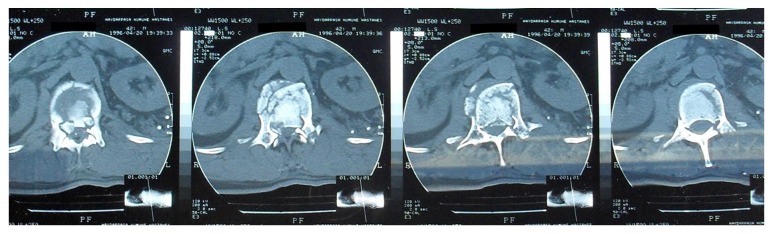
CT: fracture of both pedicles, left transverse process, and anterior and middle column of L1. There is absolutely no hemangioma or any other spinal soft tissue lesions at the left pedicle, left transverse process, and lamina.
